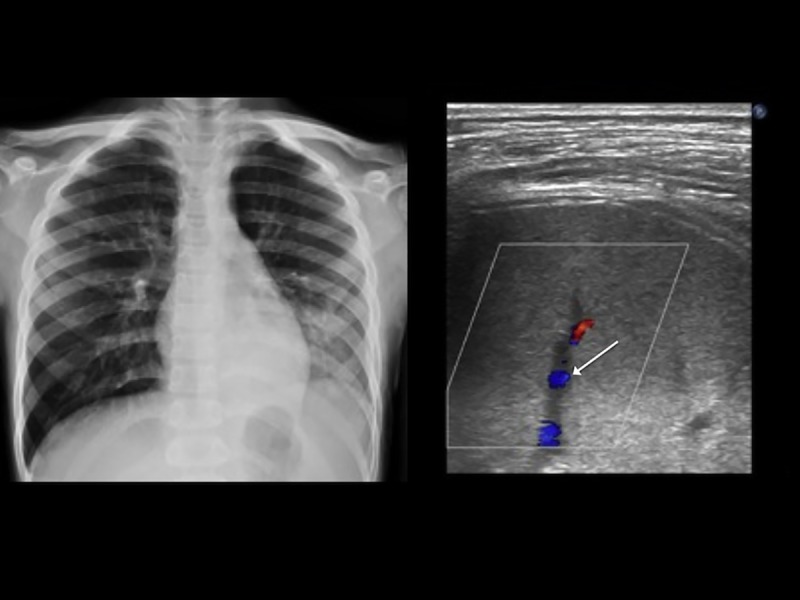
A 10-year-old boy with community acquired pneumonia. (A) Chest radiography revealed left lower lung consolidation. (B) Color Doppler sonography revealed enhanced vascularity (arrow) in the consolidation.
